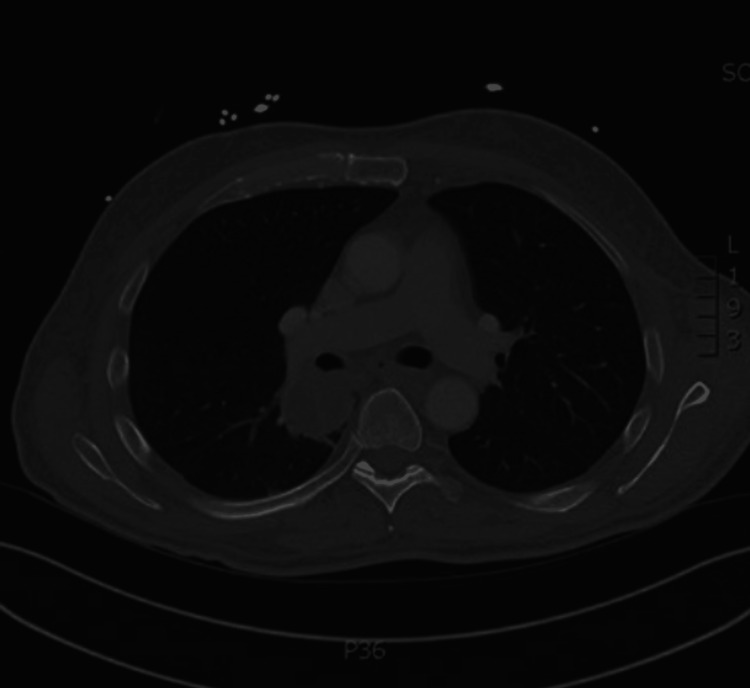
A computed tomography scan of the chest showing a large, heterogenous, predominantly right lower lobe mass measuring up to 4.3 cm with right mainstem bronchus/right lower lobe bronchus posterior wall abutment without significant luminal stenosis
